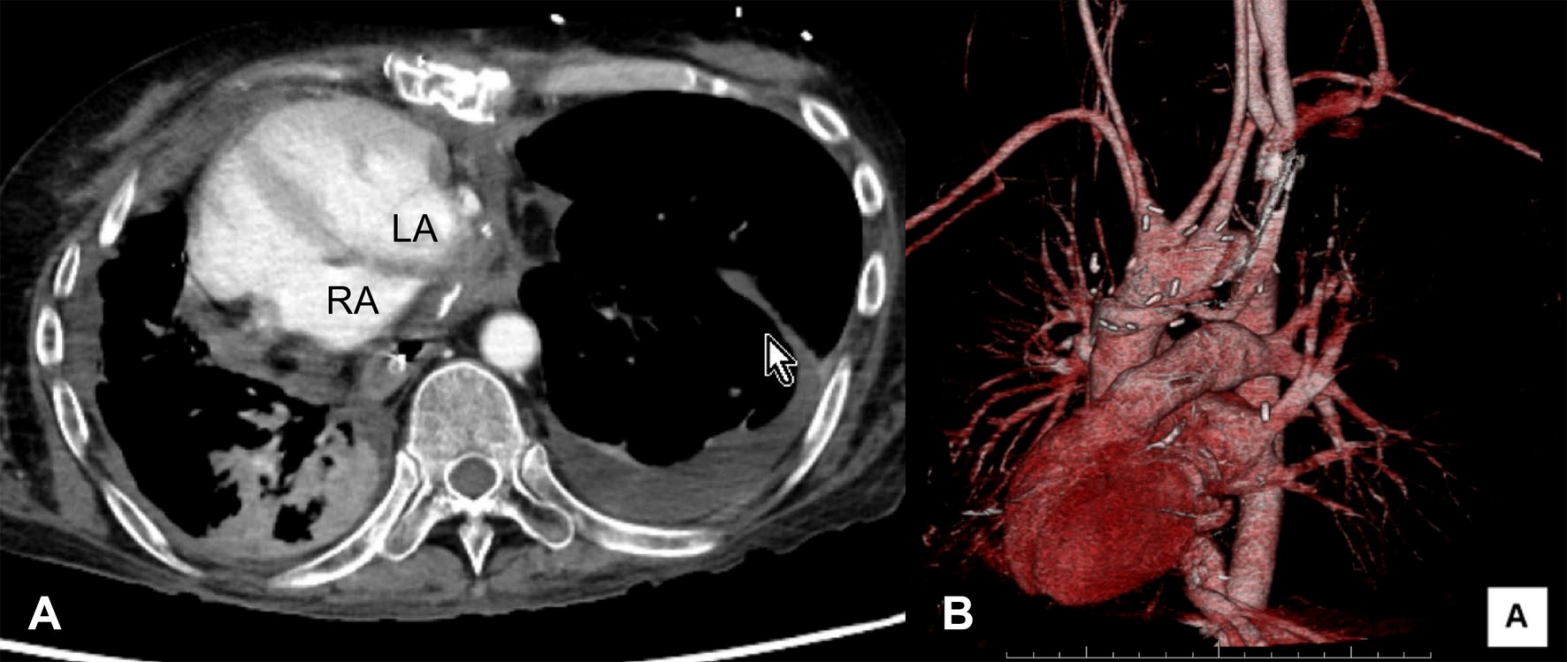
Postoperative computed tomography images: an axial image demonstrated that the transplanted heart was situated in the right hemithorax (A); a 3D image showed the heart in a dextrocardia position (B). *LA* left atrium, *RA* right atrium
